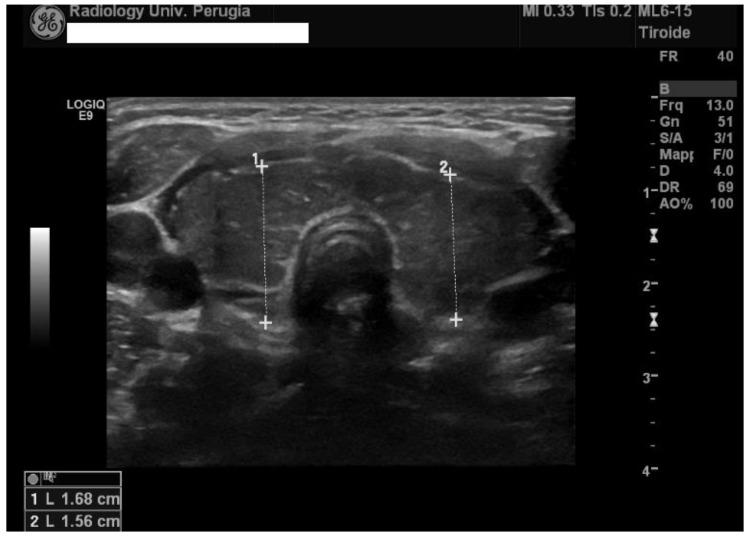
A thyroid ultrasound with evidence of an enlarged thyroid gland (total volume 10 mL) with non-homogeneous echotexture.
